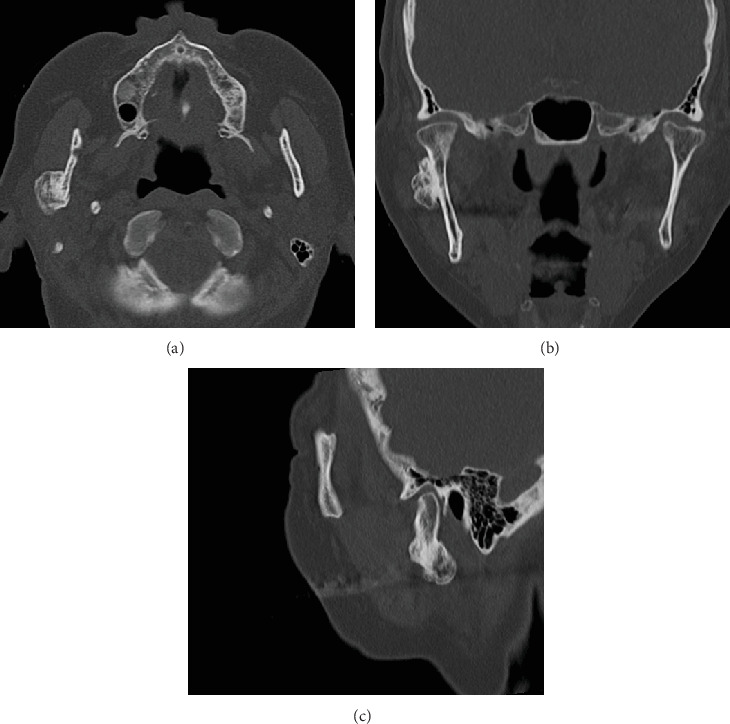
(a) Axial section of the computed tomography scan. (b) Coronal section of the computed tomography scan. (c) Sagittal section of the computed tomography scan.
